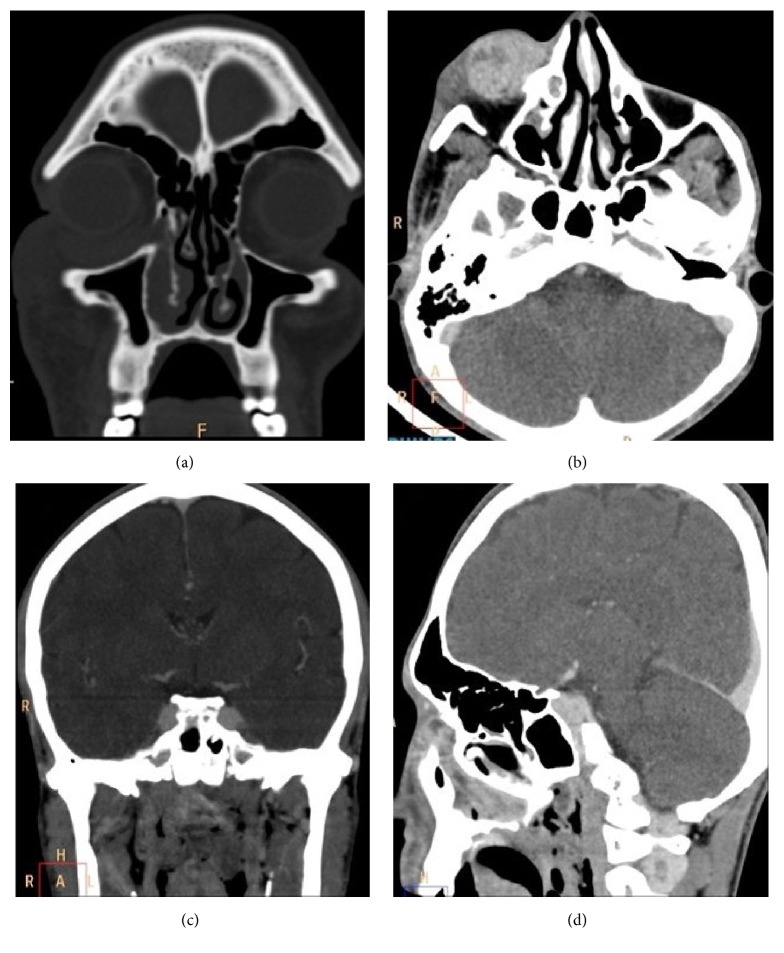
(a) Coronal image of CT paranasal sinuses in bone window showing soft tissue density mass lesion in inferior portion of right eye with extension into nasolacrimal duct. Nasolacrimal duct is expanded with rarefaction of its walls. Mass is reaching up to inferior turbinate. (b) CT postcontrast axial view in soft tissue window at the level of maxillary antrum showing well-defined, intensely enhancing mass lesion anteroinferior to right globe. (c) CT coronal image in maximum intensity projection showing abnormally hypertrophied vessels originating from nasopharyngeal mucosa and supplying the lesion. (d) CT postcontrast sagittal view in soft tissue window showing extension of the lesion through the expanded nasolacrimal duct into inferior turbinate.
